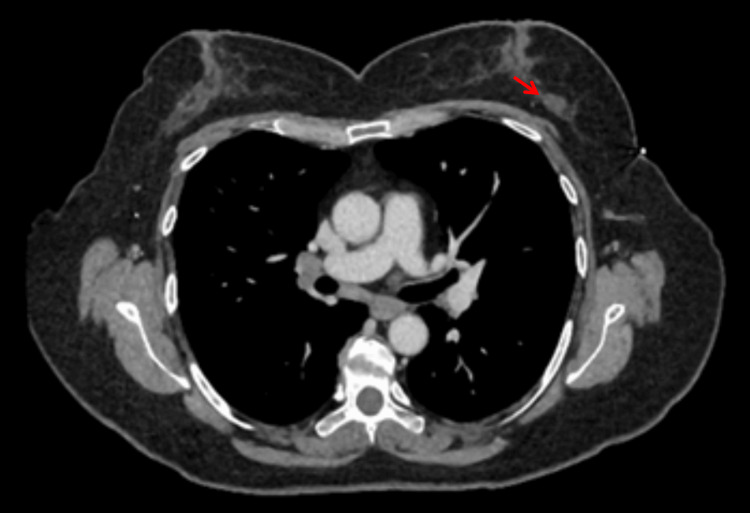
Computed tomography scan of the chest Red arrow indicates a 17-mm left outer breast nodule
